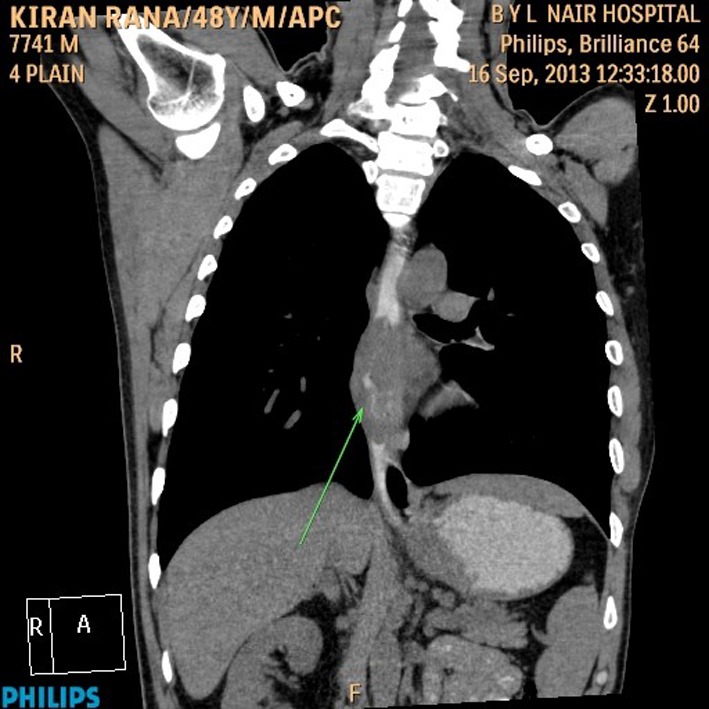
CT scan of abdomen and thorax.
